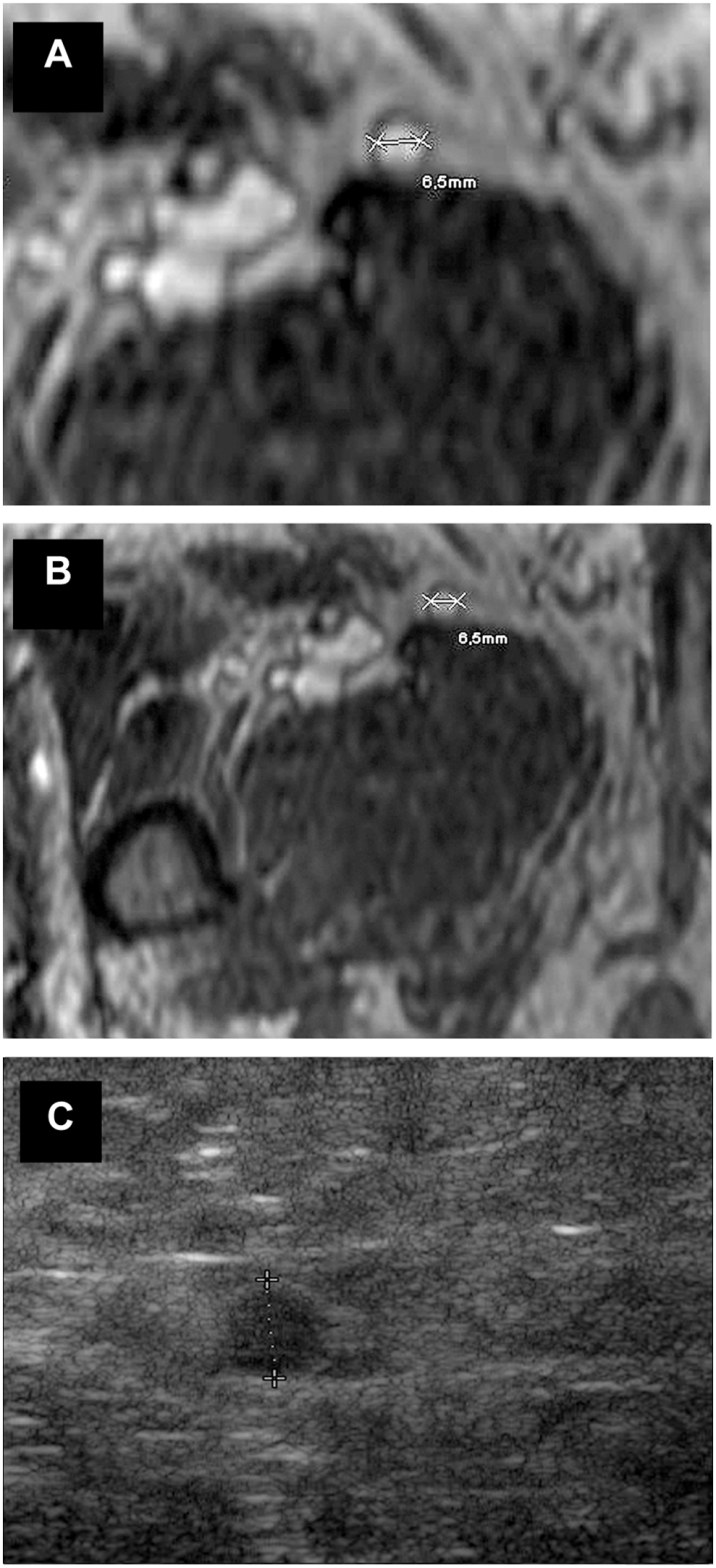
MR-angiographic and duplex sonographic images of the great saphenous vein. Magnetic resonance imaging (BPCA-MRA) and color-coded duplex sonography in the proximal level of the left GSV of a 69 year old male patient who suffered from ulcerations of the lower leg. (a, b) Axial multiplanar reformat of contrast-enhanced T1-weighted gradient-echo images during the steady-state. (c) Axial color-coded duplex sonography.
